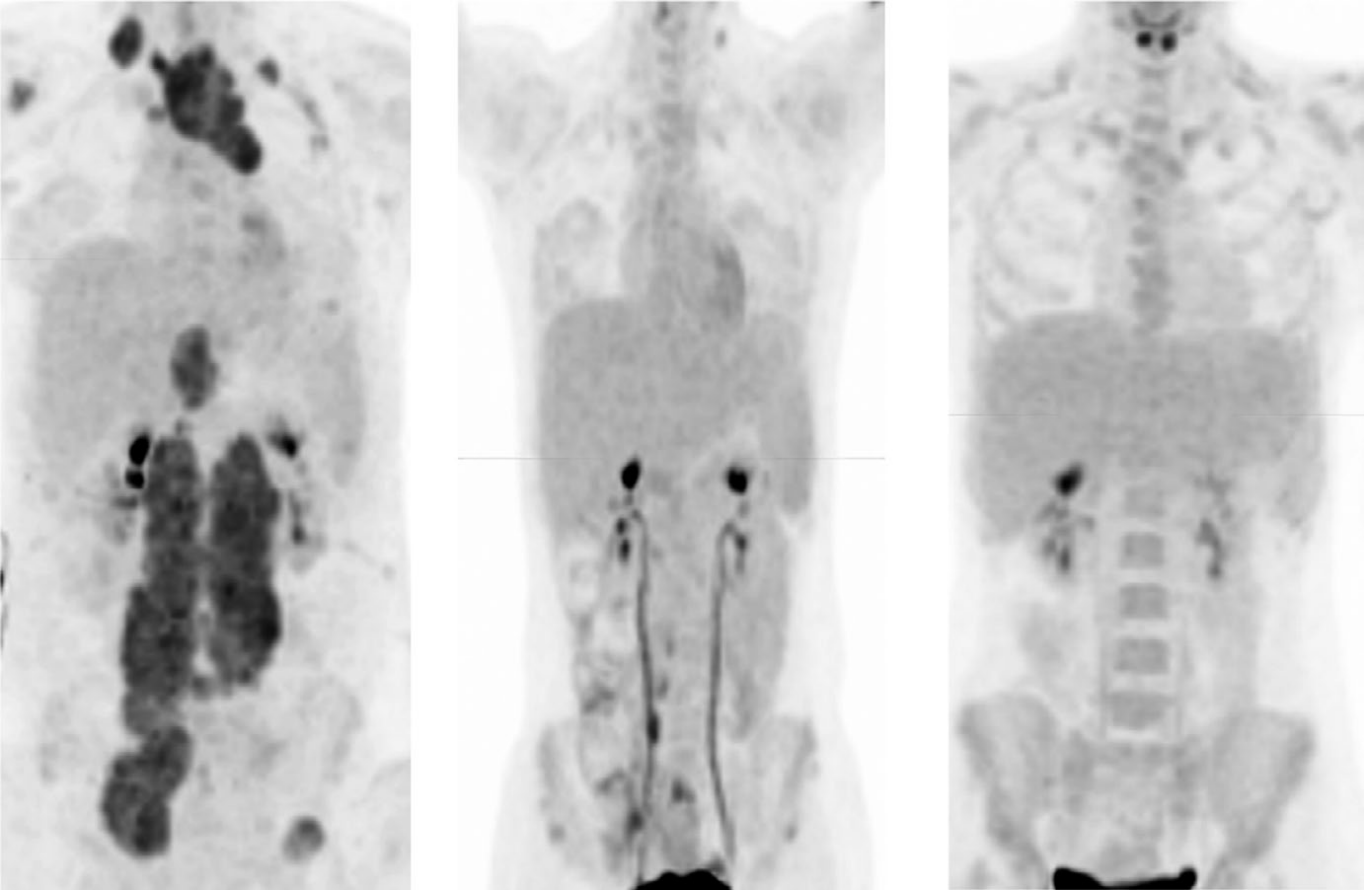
17 year old girl with alveolar RMS of the lower extremity enrolled on ARST08P1. Images at the time of presentation (left panel) show right sided supraclavicular, left anterior mediastinal, subdiaphragmatic, widespread bilateral retroperitoneal, and left iliac bone uptake. By 6 weeks most sites of uptake had resolved. SUVmax of the anterior mediastinal mass declined from 8.7 to 1.7. At 19 weeks no residual areas of abnormal uptake were present. FDG‐PET response does not correlate with EFS in either IR or HR RMS. This patient suffered distant recurrence 6 months following completion of protocol directed therapy despite excellent FDG‐PET response, and eventually died from recurrent disease
